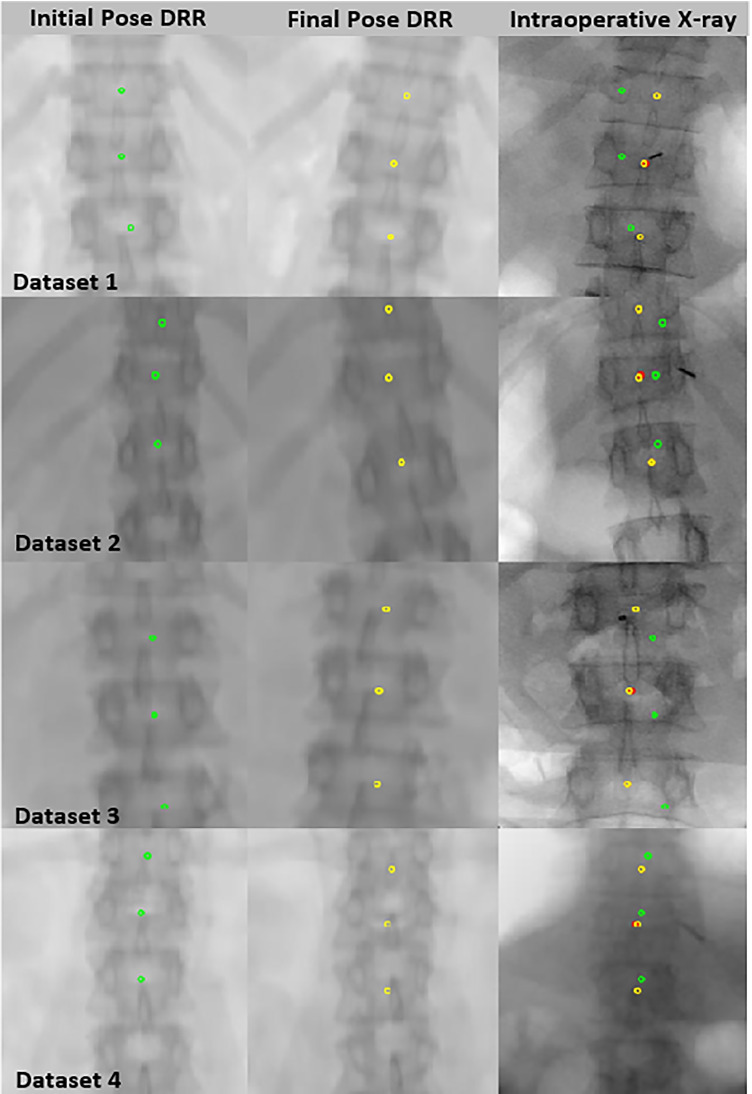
Overlay of vertebral centroids from pCT image on DRR image at initial pose (column 1, green), final pose (column 2, yellow), and on intraoperative X-ray image (column 3, red)
